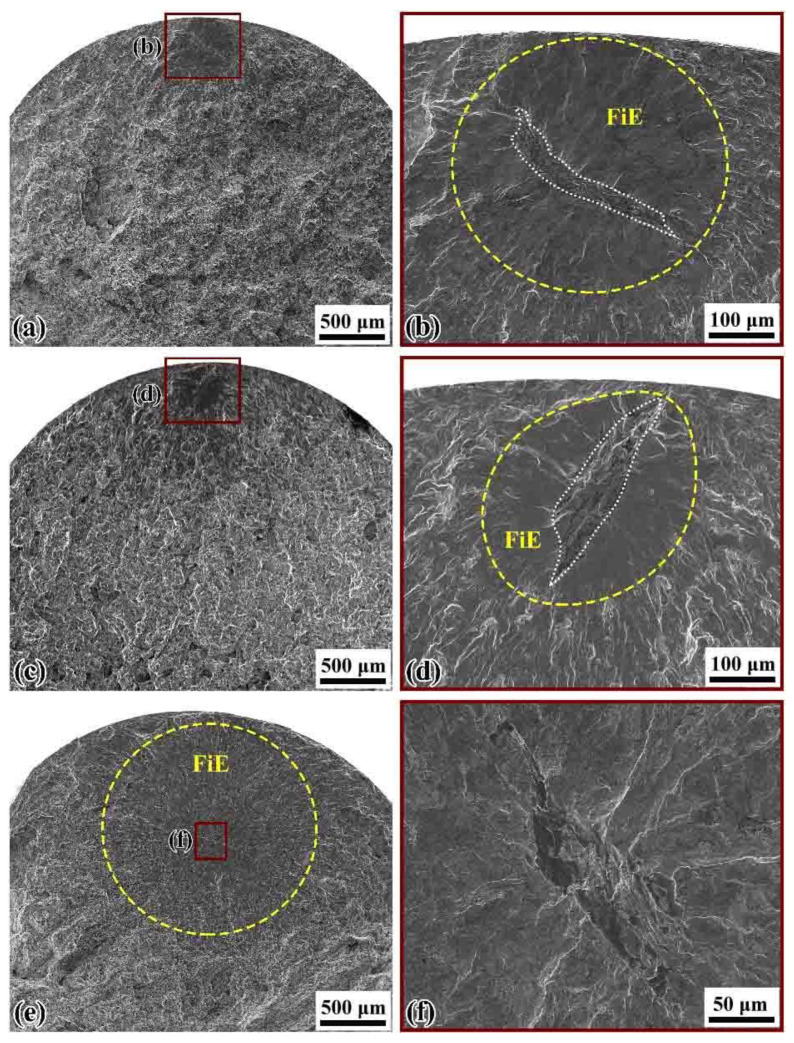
Typical fracture morphologies of internal crack initiation by ultrasonic fatigue test: (a) *R* = −1, *σ*_a_ = 262 MPa, *N*_f_ = 7.78 × 10^6^ cycles; (c) *R* = −1, *σ*_a_ = 258 MPa, *N*_f_ = 1.01 × 10^7^ cycles; (e) *R* = 0.3, *σ*_a_ = 202 MPa, *N*_f_ = 1.84 × 10^7^ cycles; while magnified views of inclusions inducing final failure are shown in (b,d,f), respectively. FiE: “fish-eye” morphology.
